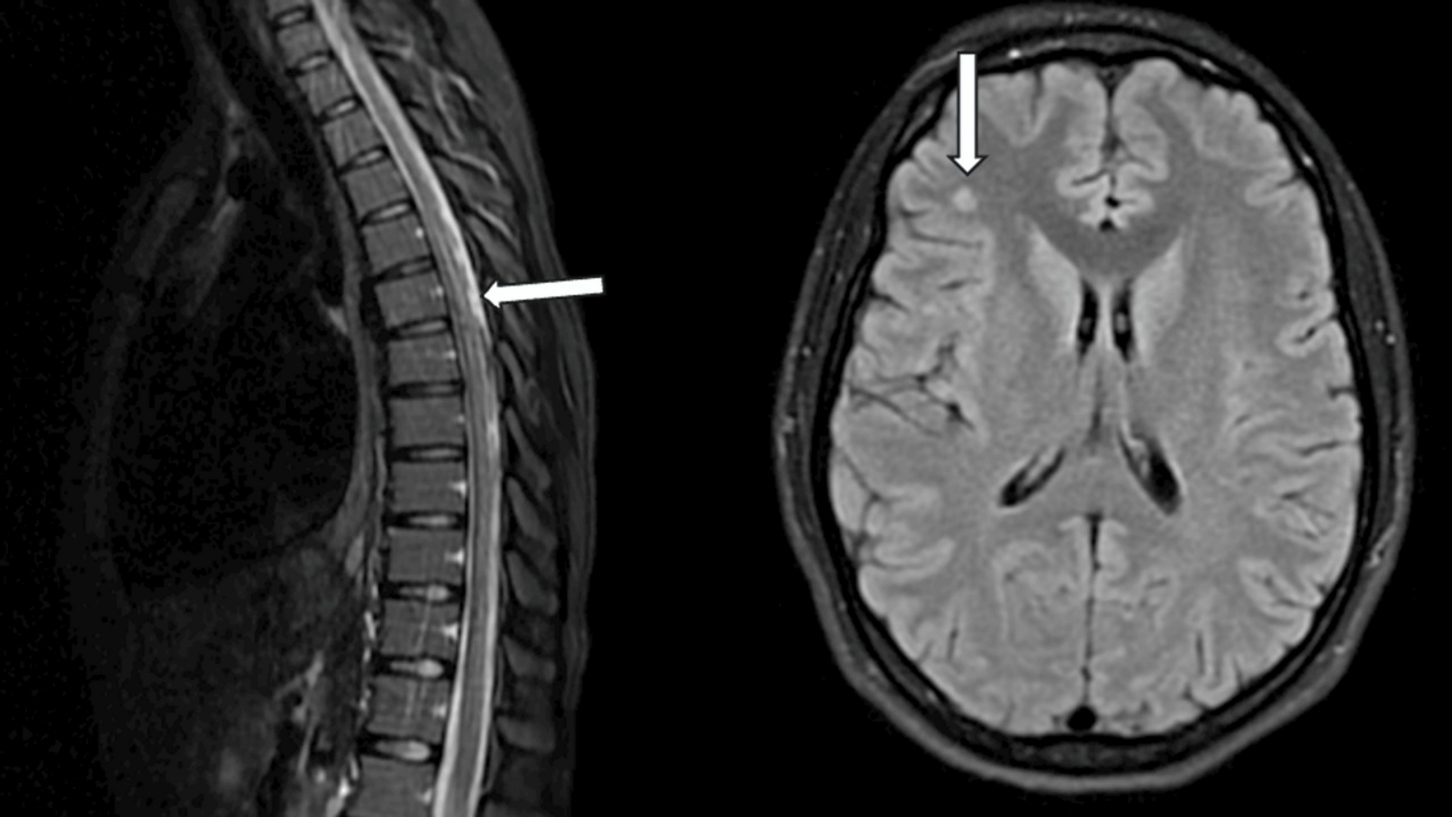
Nitrous oxide-induced myelopathy. T2-weighted image shows hyperintensities longitudinally in the dorsal column of the thoracic spinal cord, sagittal view (left). T2-weight hyperintense signal in the white matter of the right frontal lobe (right).
